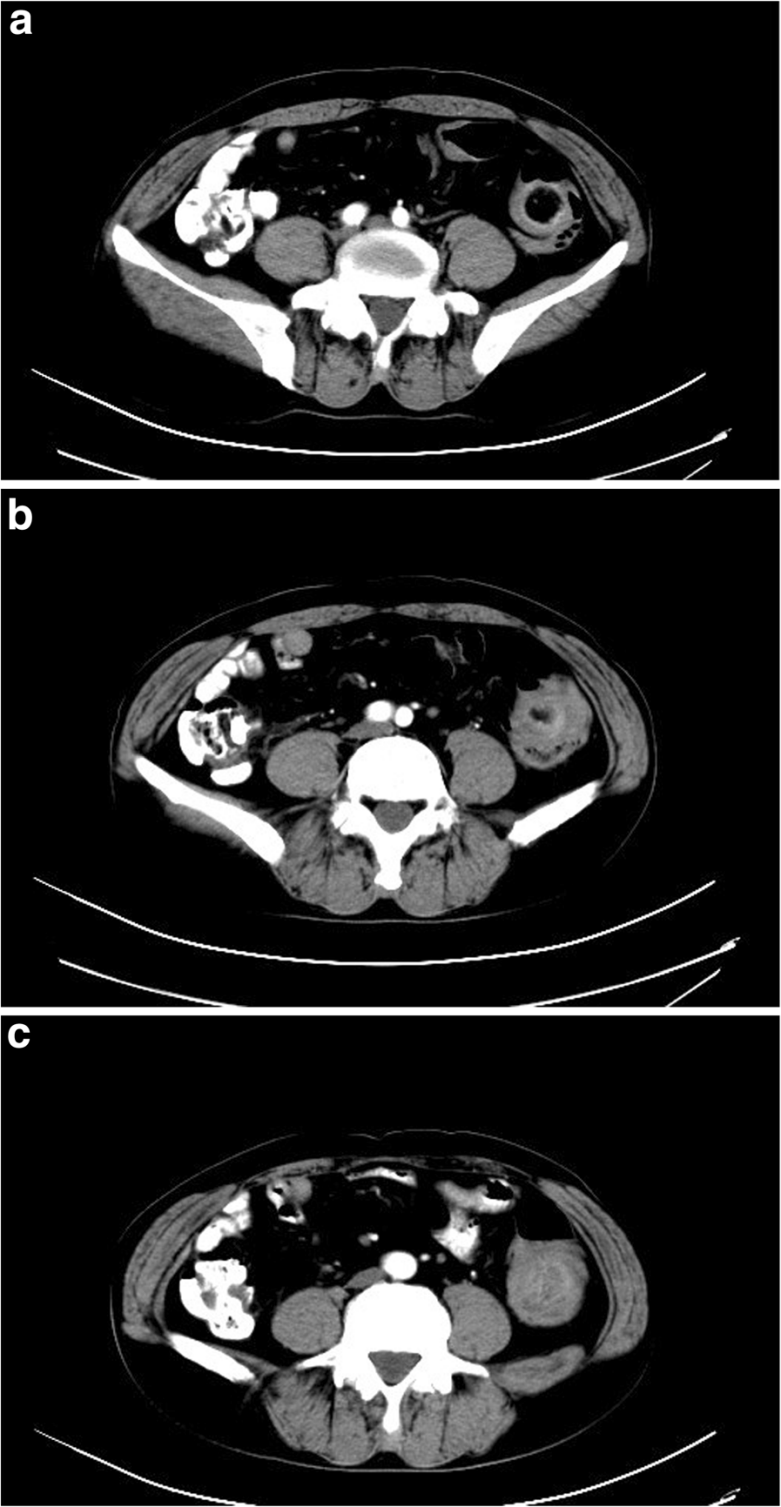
Abdominal CT scan with contrast from (a) to (c) showed a 5 cm diameter mass-like lesion at the junction of the descending and sigmoid colon with significantly thickened walls with dilated lumen. There were multiple rings within the mass with smooth margins suggesting colon intussusception. CT, computed tomography.
